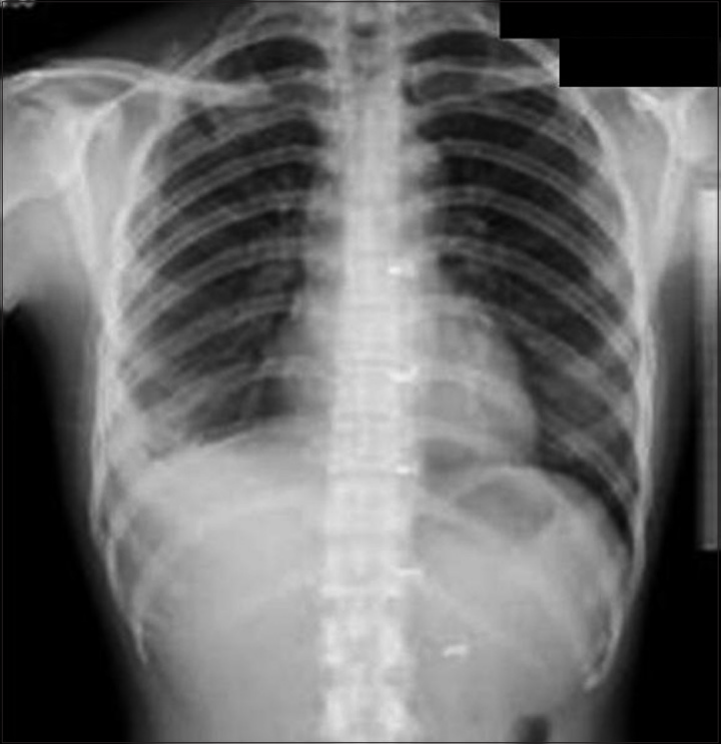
Pneumocystis carinii* pneumonia atypical features. Chest X-ray shows diffuse, vague, ill-defined lung nodules associated with a right-sided pleural effusion (confirmed by ultrasound)
